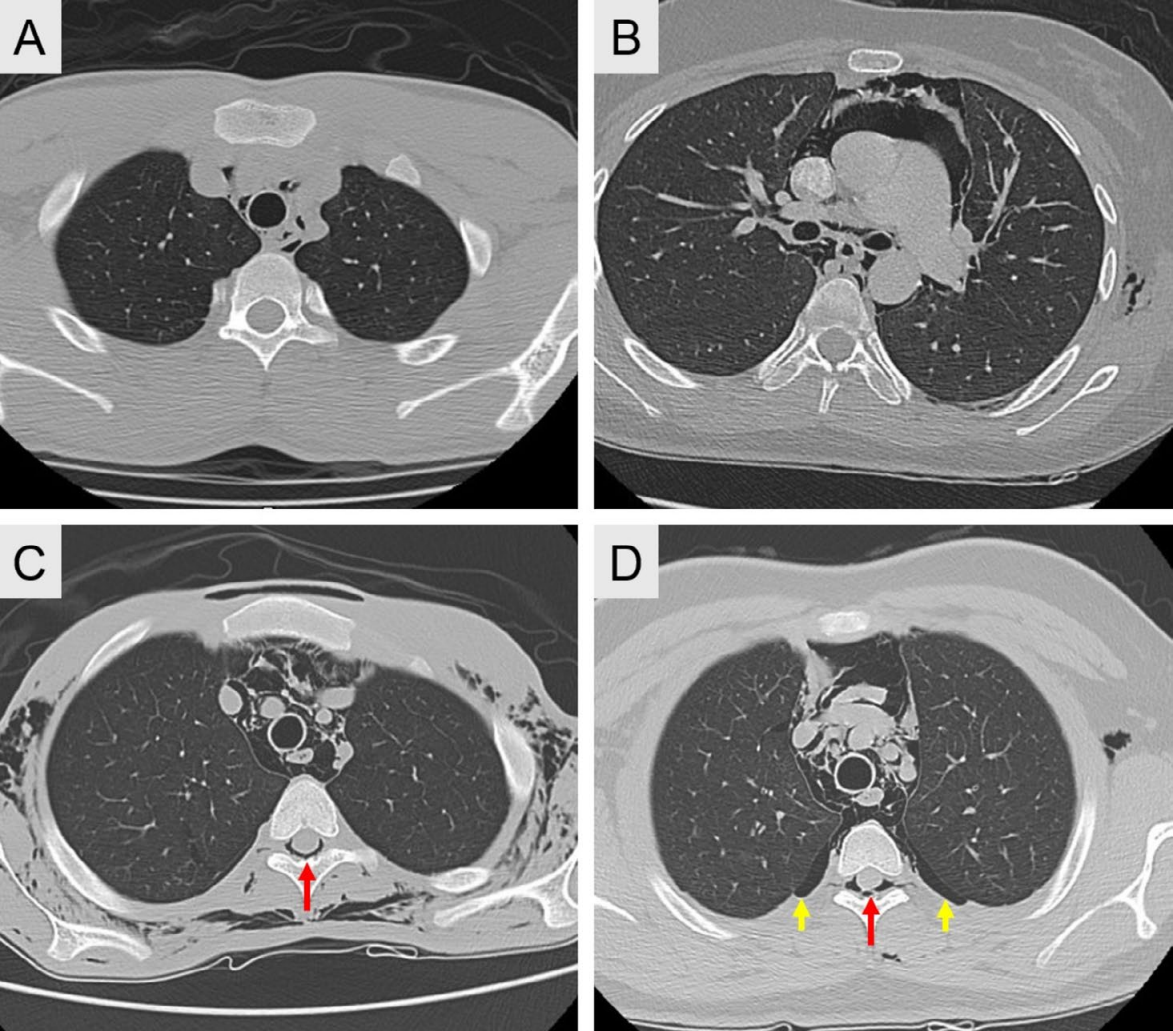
Representative computed tomography (CT) images of spontaneous pneumomediastinum (SPM) cases, classified by severity criteria. (A) Mild (Grade A): Minimal mediastinal air extending from the larynx to the carina, with limited cervical subcutaneous emphysema. (B) Moderate (Grade B): Features of mild SPM plus involvement of the peribronchial tree and pericardial region, along with subcutaneous emphysema. (C) Severe (Grade C): Features of moderate SPM accompanied by extensive chest wall subcutaneous emphysema and coexisting pneumorrhachis (indicated by red arrow). (D) SPM with concurrent subcutaneous emphysema, pneumorrhachis (*red arrow*), and pneumothorax (*yellow arrow*). SPM, spontaneous pneumomediastinum.
